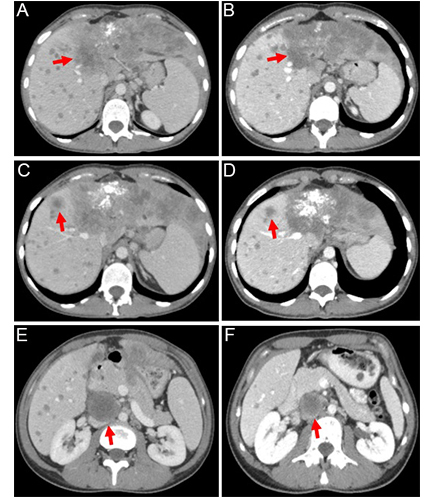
Contrast-enhanced abdominal computed tomography (CT) scans were performed at baseline (left) and after 2 months of the triple-agent therapy (right). The treatment led to a reduction in the size of the following lesions (marked by red arrows): the tumor located in S4 of the liver [from 147 mm (A) to 115 mm (B); a decrease of 22%], the lesion in S8 of the liver [from 20 mm (C) to 17 mm (D); a decrease of 15%], and the conglomerate between the head of the pancreas and the inferior vena cava [from 42 mm (E) to 35 mm (F); a decrease of 17%]
